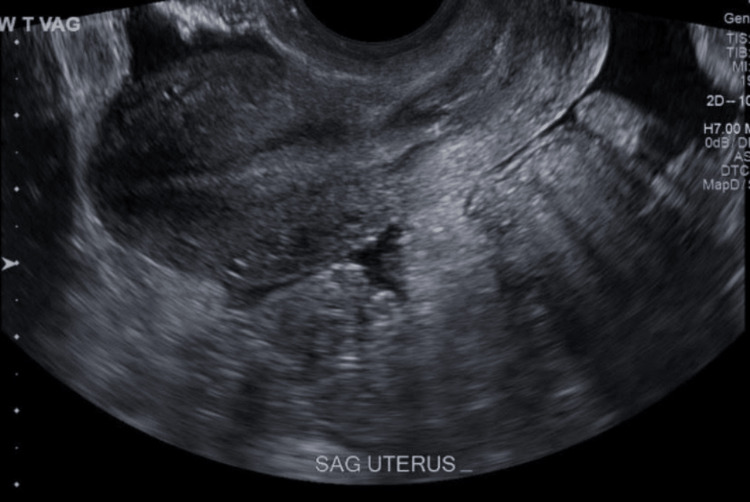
Transvaginal ultrasonogram. The longitudinal image revealed the uterine cavity with no intrauterine pregnancy.
